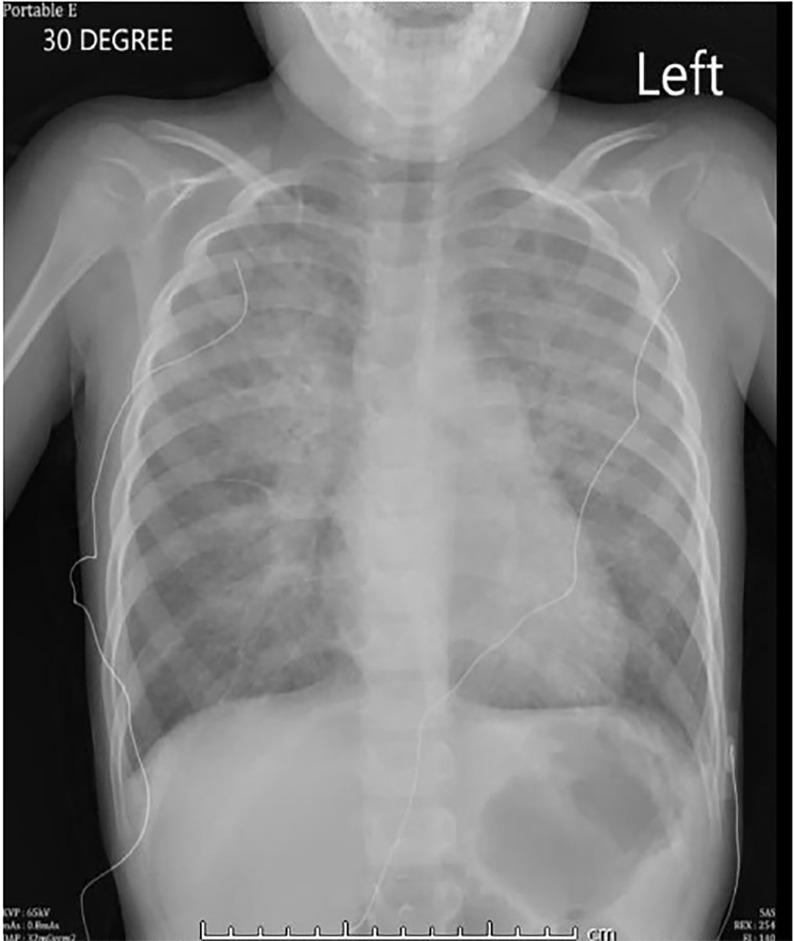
Initial chest radiograph demonstrating pulmonary edema.
